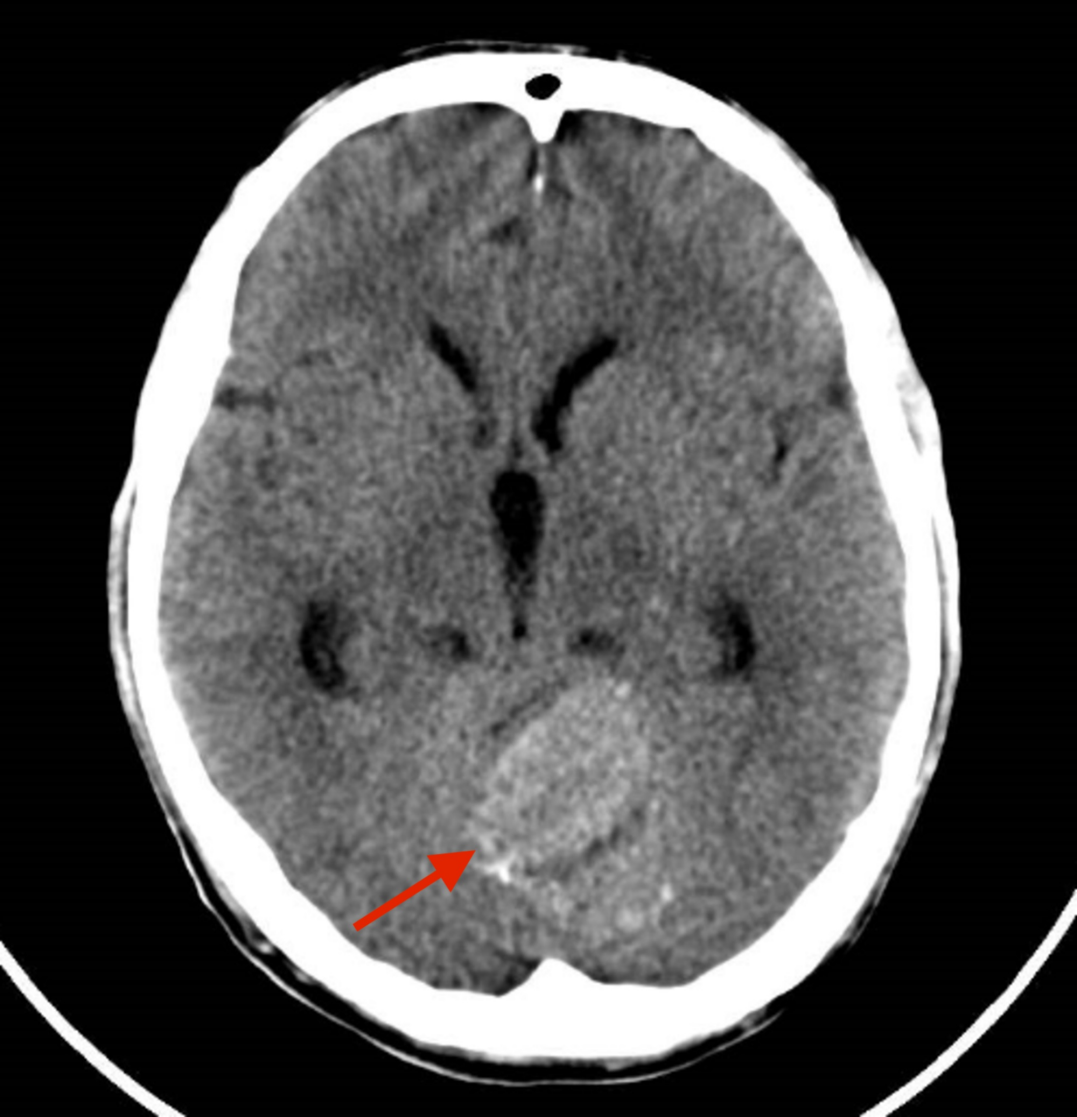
Computed tomography showing bleeding of the central nervous system.
